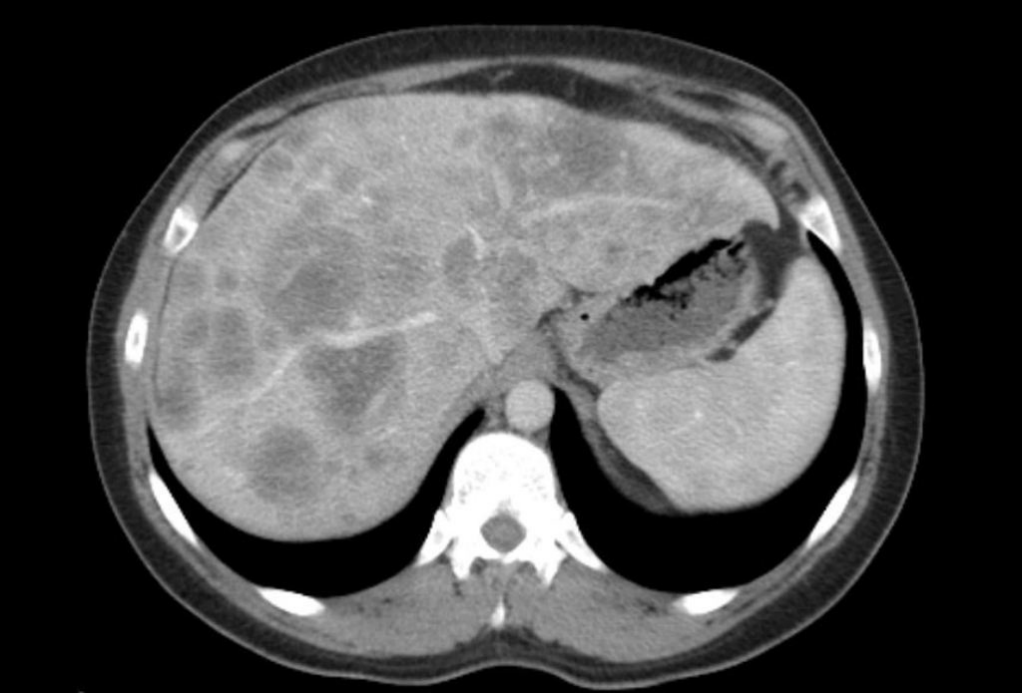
Liver metastases (computed tomography)
